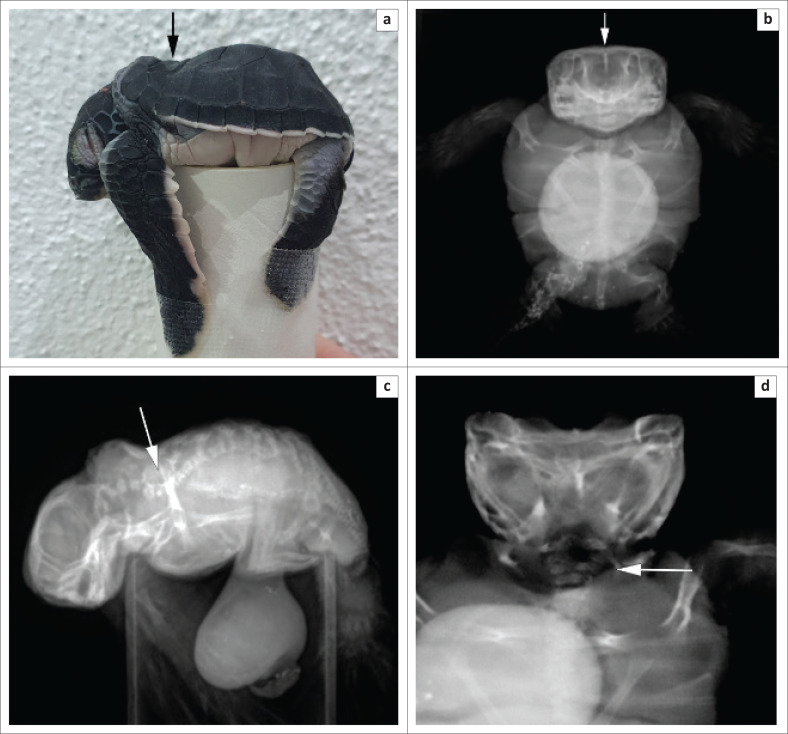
Radiographic images of the malformed hatchling. (a) Positioning of the hatchling for lateral image acquisition – spinal deformation clearly visible (arrow). (b) Ventral view – yolk sac visible and fusion of the two skulls indicated by arrow. (c) Lateral view – lordotic region of the spin indicated by arrow. (d) Dorsal view – point of fusion of the two necks indicated by arrow.
